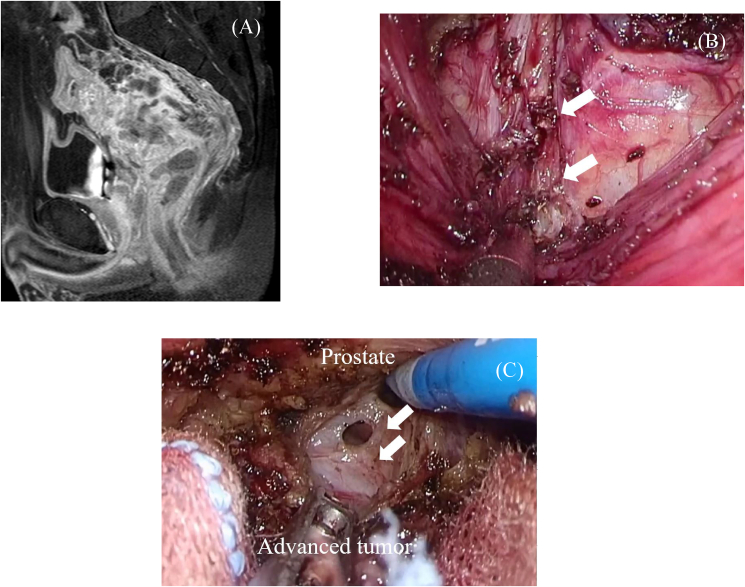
Preoperative and intraoperative findings in a patient who underwent hybrid surgery. (A) Preoperative magnetic resonance imaging after neoadjuvant treatment. (B) Dissection of the hiatal ligament (arrows) and below the endopelvic fascia of the distal tumor to secure the circumferential resection margin. (C) Dissection of Denonvilliers' fascia (arrows) to secure the circumferential resection margin.
